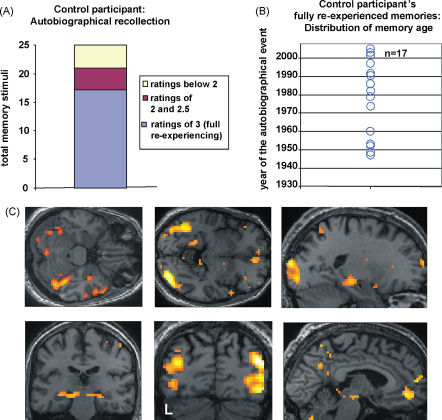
Control participant: recollection of autobiographical memories. (A) The bar in this graph represents the total number (25) of autobiographical memory photograph stimuli used in the scanning experiment. The legend beside the graph indicates the share of the rating types. See also Table 2. (B) A plot showing those memories that were fully recollected and re-experienced by the control participant, with the year the event happened shown on the *y*-axis. *N* = 17 for fully re-experienced memories – note that some data points overlap C. The brain network supporting retrieval of autobiographical memories in the control participant. See Table 3 for full details of activation locations. Views of this distributed brain network are shown (*p* < 0.005 uncorrected) on a selection of relevant axial, sagittal and coronal sections from the control participant's structural MRI. L: left side of the brain.
